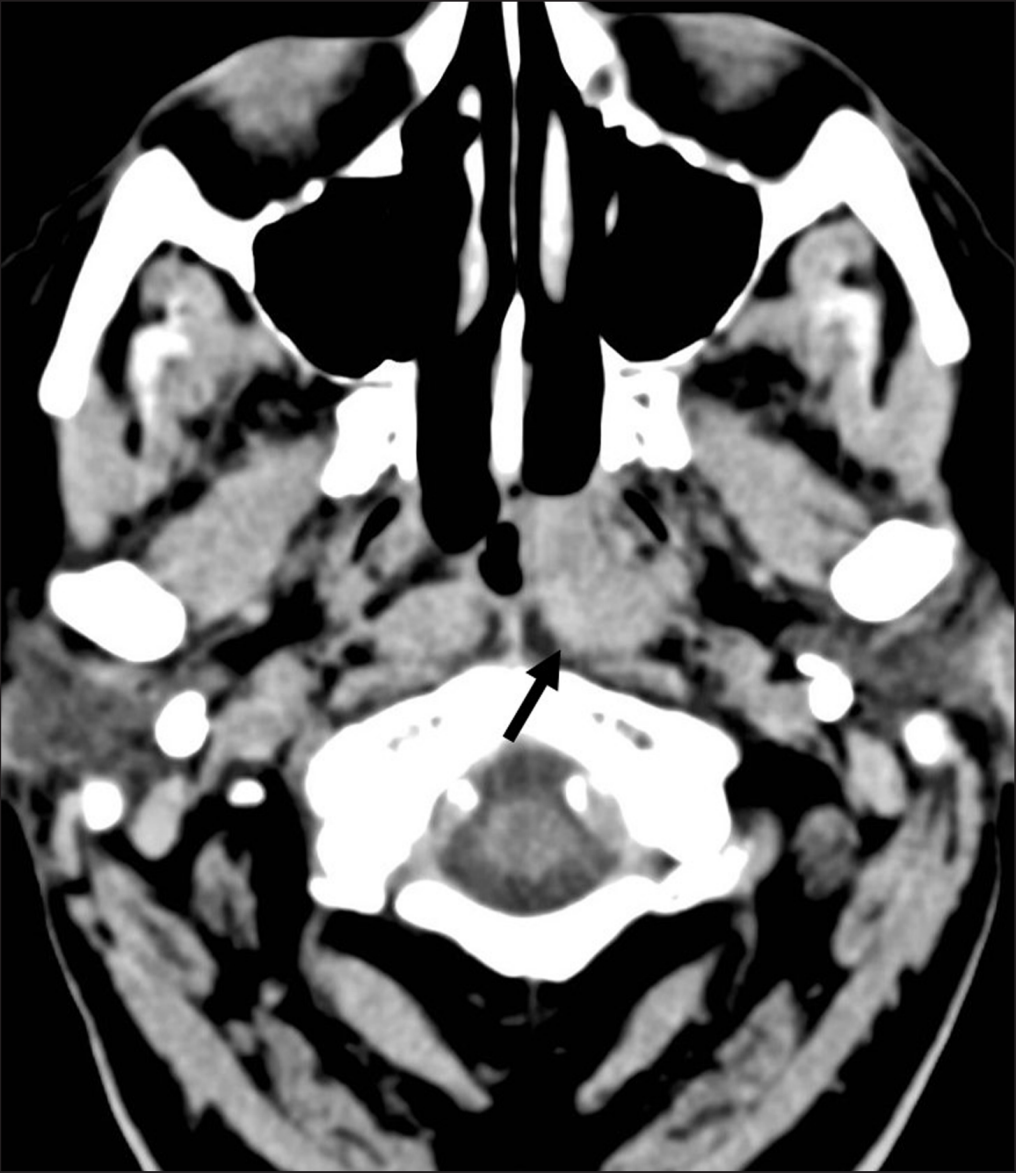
The lesion was pear-shaped and well circumscribed. The lesion was slightly hyperintense on T1-weighted images (WI) (Figure 2A, white arrow) and hyperintense on fat-suppressed T2-WI (Figure 3, white arrow). There was subtle peripheral enhancement after administration of gadolinium contrast (Figure 2B, white arrow). The diagnosis of a retention cyst of Rosenmüller’s fossa was made. As the patient was asymptomatic, watchful waiting was recommended.
